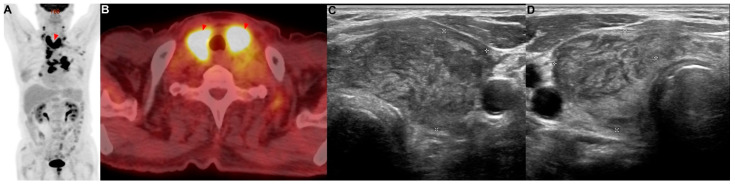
Patient 2: a 77-year-old man diagnosed with squamous cell carcinoma of the lung with metastases in the thyroid. (A,B) PET/CT showed metastatic lesions (red arrowhead) in the bilateral lobes of the thyroid. (C,D) Metastatic lesions were observed in the (C) left and (D) right thyroid.
